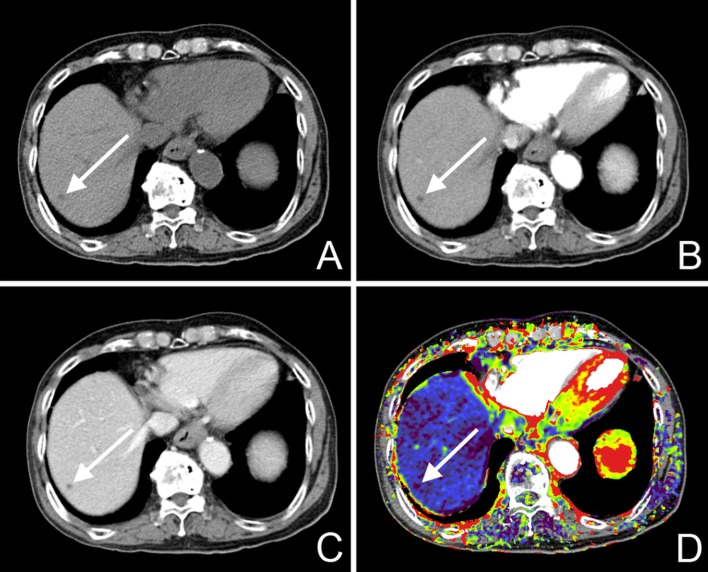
A 64-year-old female patient with sigmoid colon cancer showed a tiny low-attenuating lesion in the S8 of the liver on a dynamic CT scan (A–C). Because this lesion showed no interval change for 2 years on follow-up imaging studies and presented as a high SI lesion on T2-weighted MR images, it was diagnosed as a hepatic cyst. The AEF color map presented the lesion as a nodule (arrow) with decreased AEF (D).
